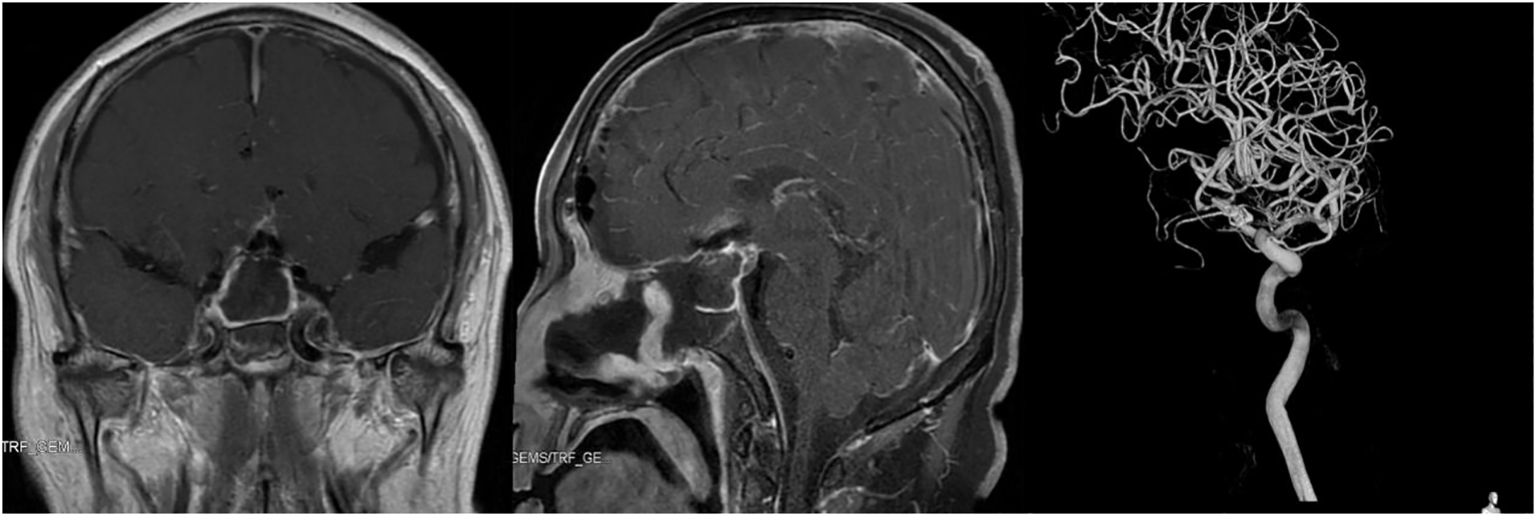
Imaging examination of the reported patient after operation. Left and Middle: Pituitary enhanced MRI showed complete removal of sellar region lesion 3 days after operation. Right: Catheter-based cerebral angiography indicated no obvious abnormality after clipping of anterior communicating artery aneurysm.
